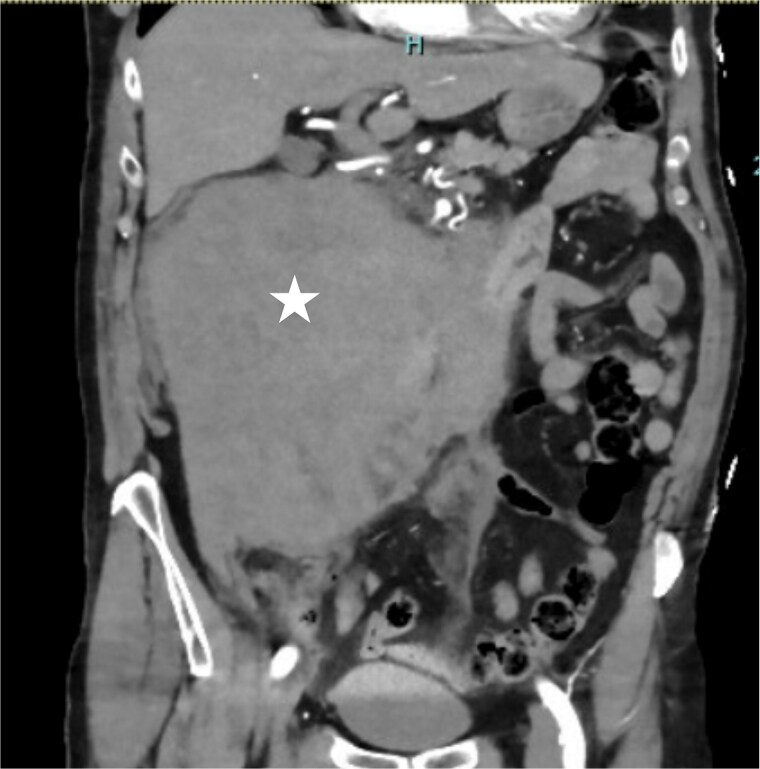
Coronal contrast-enhanced CT demonstrating a massive right-sided retroperitoneal hematoma (asterisk).
